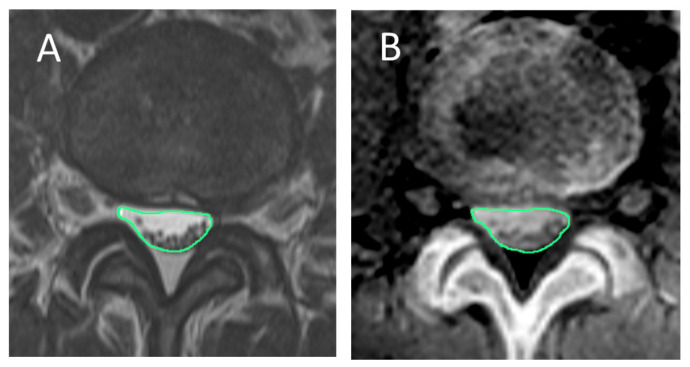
Measurement of spinal canal area. On the same axial cross-sections of both (A) T2-weighted image and (B) CT-like image, the spinal canal area was measured by manually tracing along the inner border of the dura mater.
